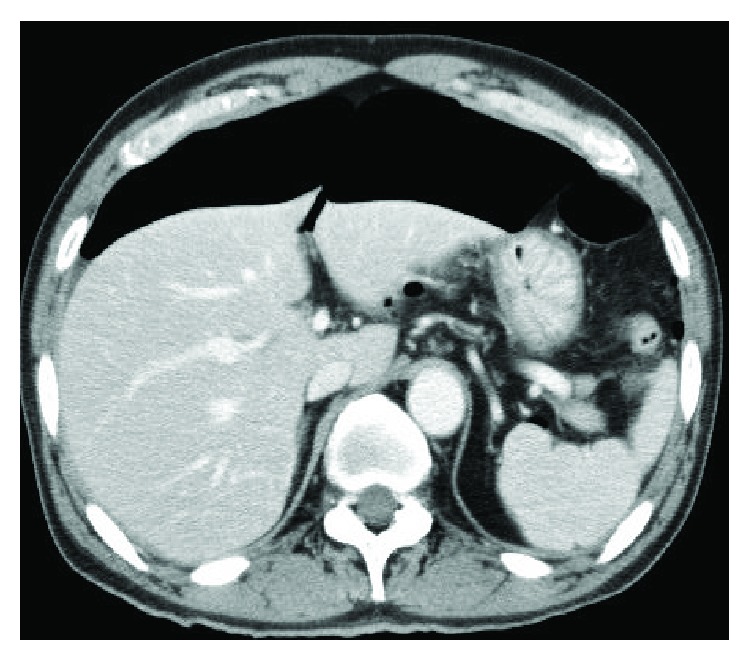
Emergent CT shows massive free air when the patient complained of severe abdominal pain.
